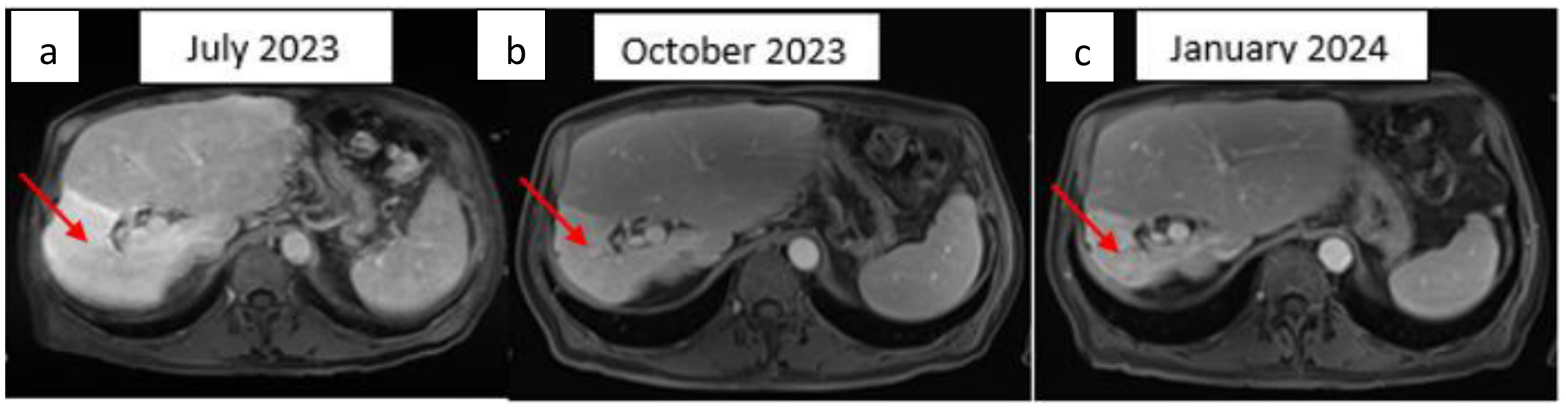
Hepatic T1 MRI image after gadolinium injection for three min. Axial image: (A) Bilocular hepatic tumor with an encapsulated appearance in segment VI of the right liver, initially measuring 69 × 68 × 81 mm. (B) Partial response with a 25% reduction after three months of ICI therapy. (C) Partial response with 50% reduction mm after six months of ICI therapy. The red arrow indicates the hepatic tumor.
